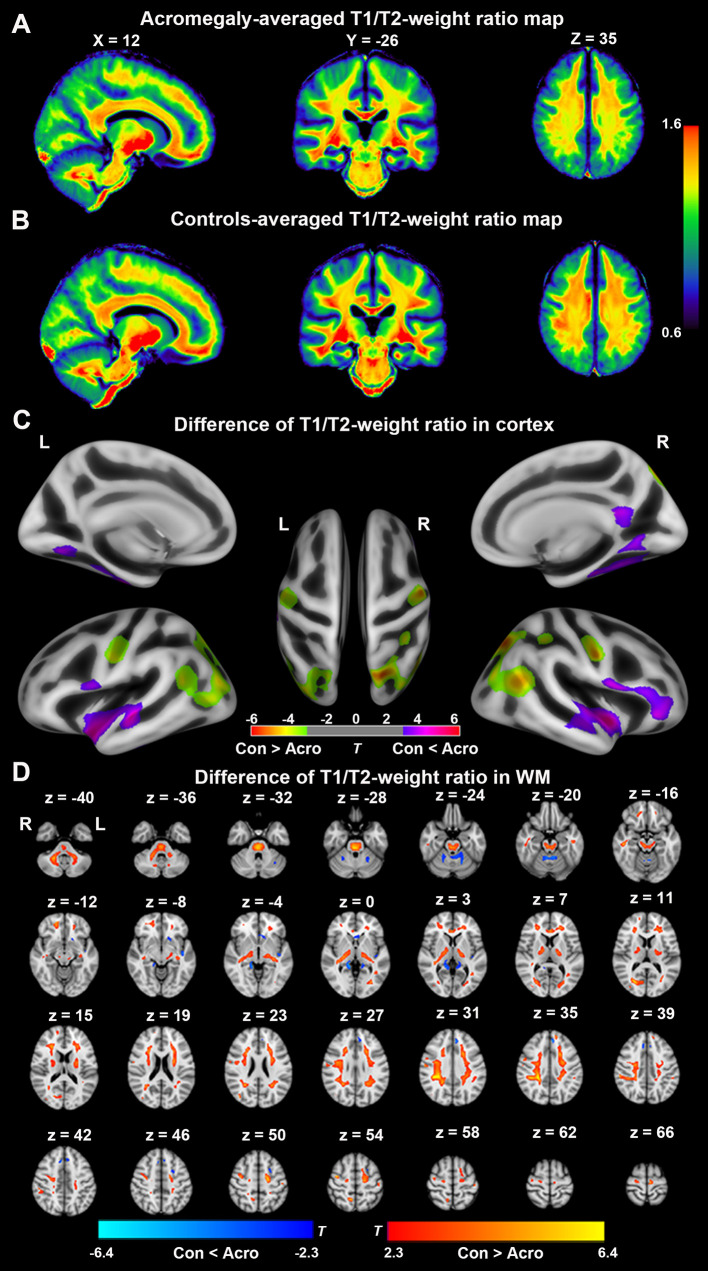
Difference of T1/T2-weight ratio in cortex and white matter (WM) between acromegaly and controls. Average T1/T2-weighted ratio maps from acromegaly (A) and controls (B). (C) Green-yellow indicates the T1/T2-weight ratio in cortex was significantly decreased in acromegaly compared to controls (pFDR < 0.05). Violet-red indicates the T1/T2-weight ratio in GM was significantly increased in acromegaly compared to controls (pFDR < 0.05) (D) Red-yellow indicates the T1/T2-weight ratio in WM was significantly decreased in acromegaly compared to controls (pFDR < 0.05). Blue indicates the T1/T2-weight ratio in WM was significantly increased in acromegaly compared to controls (pFDR < 0.05). Con, controls; Acro, acromegaly.
